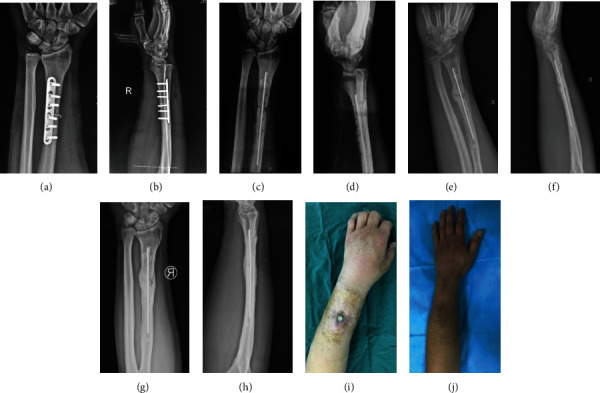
X-ray 3 months after internal fixation of the radial fracture (a, b), after Kirschner wire open placement of bone cement (c, d), at 3 months (e, f), and at 1 year (g, h) postoperatively. General images before (i) and after (j) treatment.
